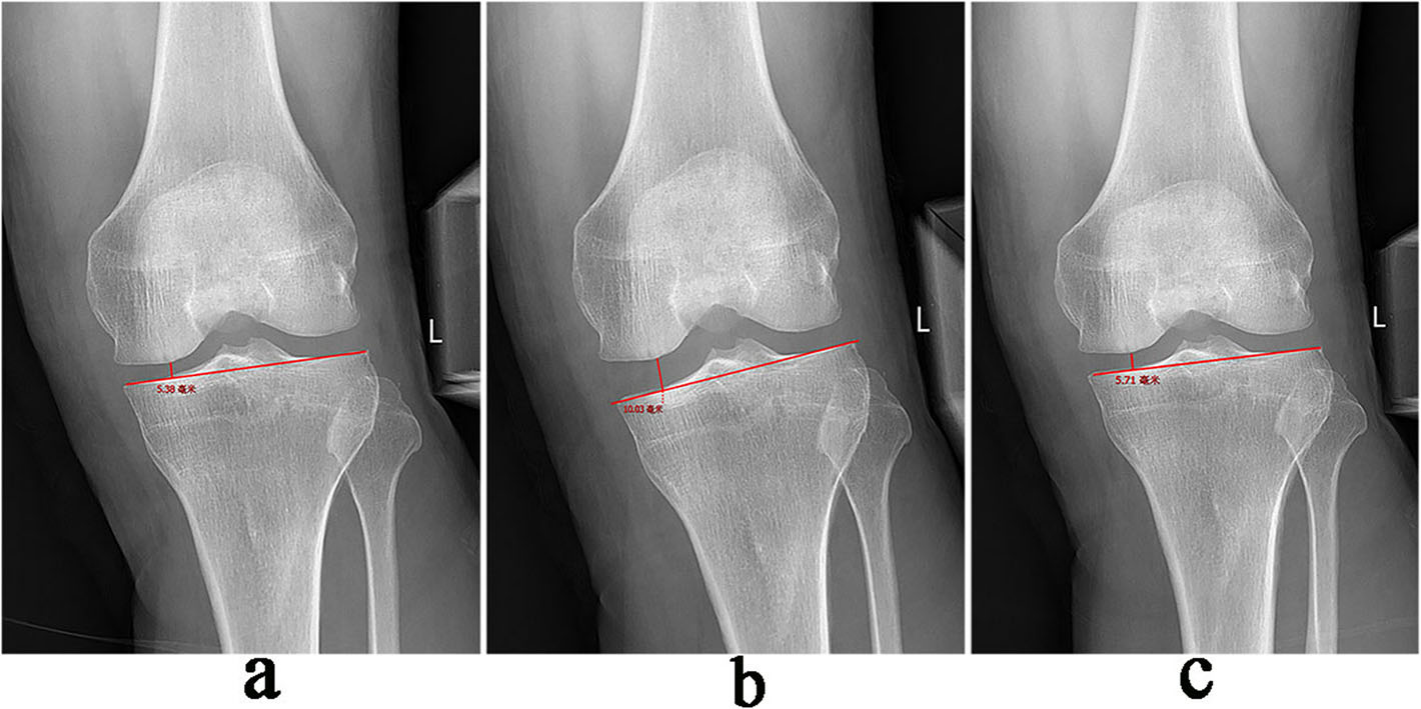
Radiographic measurement of JSW under valgus stress radiograph. The distance of JSW (perpendicular line) was measured using PACS software. a The JSW was 5.38 mm preoperatively. b 10.03 mm in the 1st week postoperatively. c 5.71 mm by the 3rd month postoperatively
